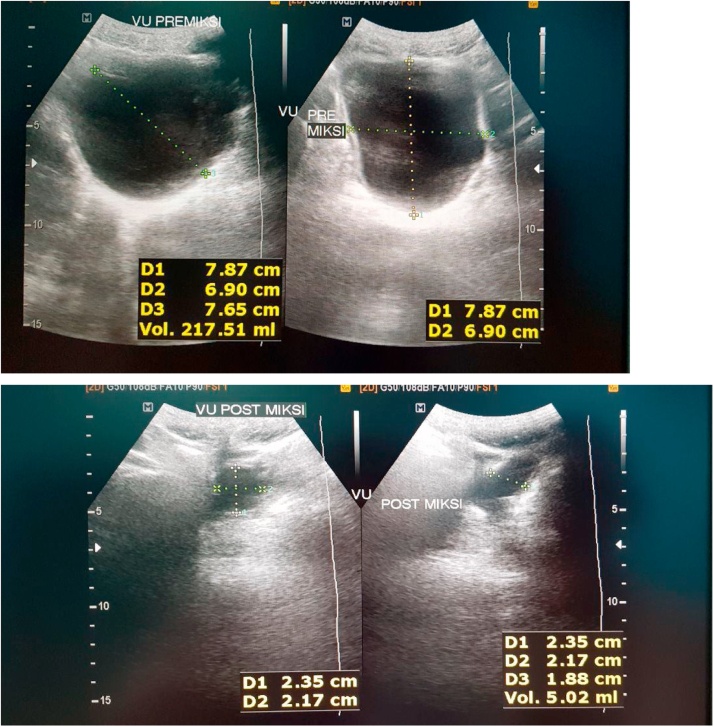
Pre-micturition and post-micturition ultrasound of post-bladder augmentation patient (3 years after surgery).
